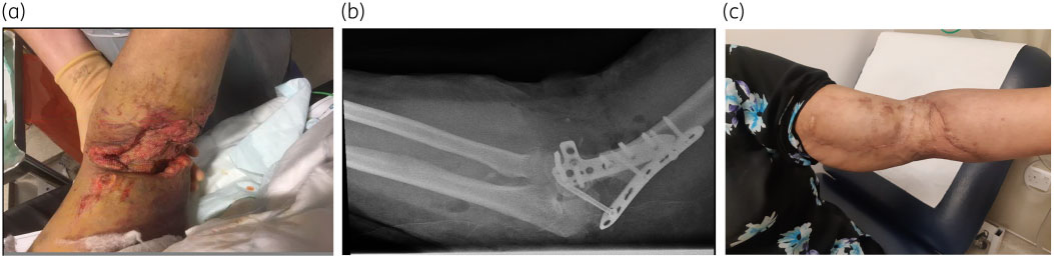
(a) Open wound in left arm at time of presentation. (b) Plain X-ray of left arm at time of presentation. (c) Left elbow after completion of antimicrobial therapy.
